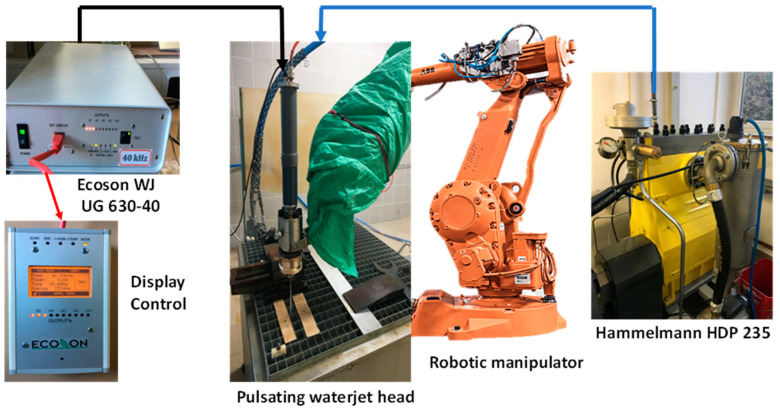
Overview of experimental setup for ultrasonic pulsating water jet composed from ultrasonic generator feeding the converter utilizing the inverse piezoelectric effect, causing the mechanical oscillation of the sonotrode in acoustic chamber.
